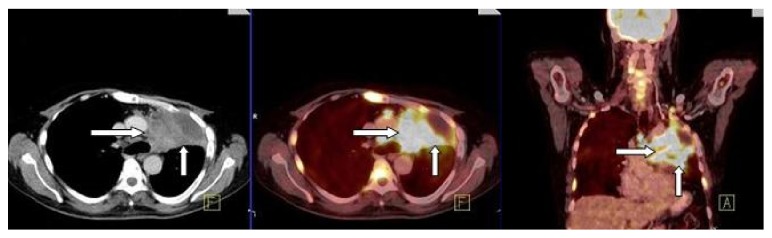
Second PET/CT: axial CT, axial PET/CT, and coronal PET/CT images revealed an increase in the size and metabolic activity of the primary mass in the left lung and mediastinal lymph nodes (arrows) when we compared with first PET/CT images.
